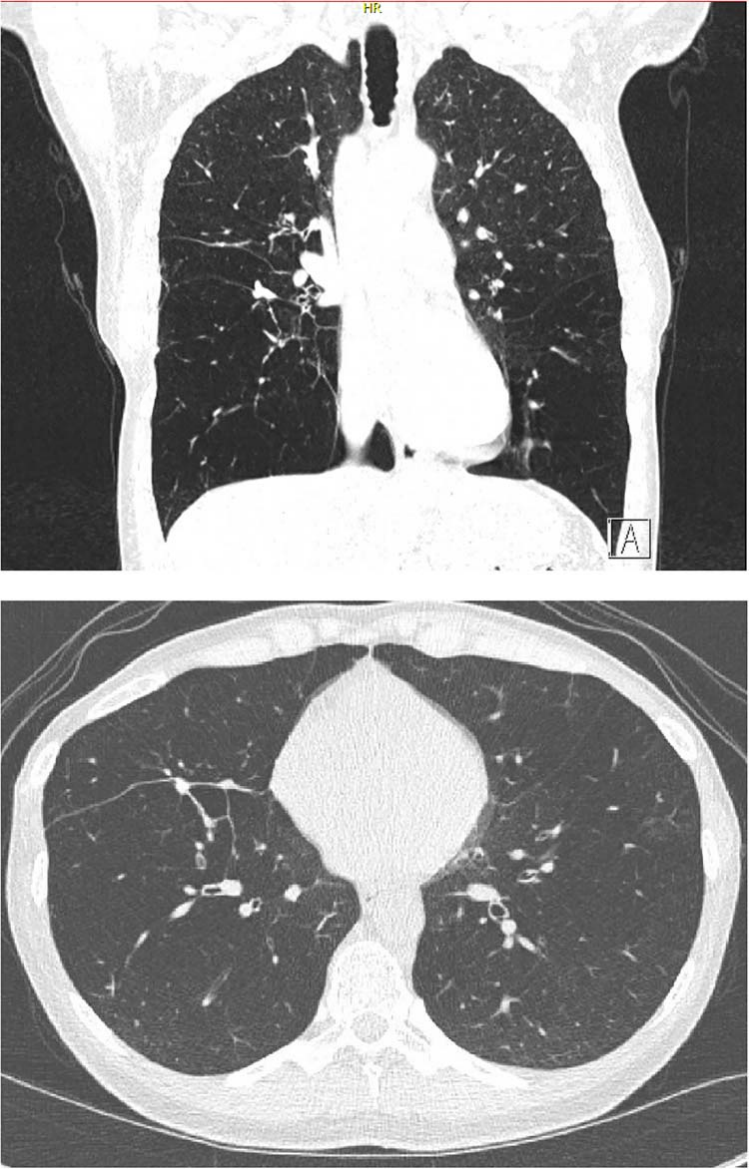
Coronal and axial sections of the chest CT.
